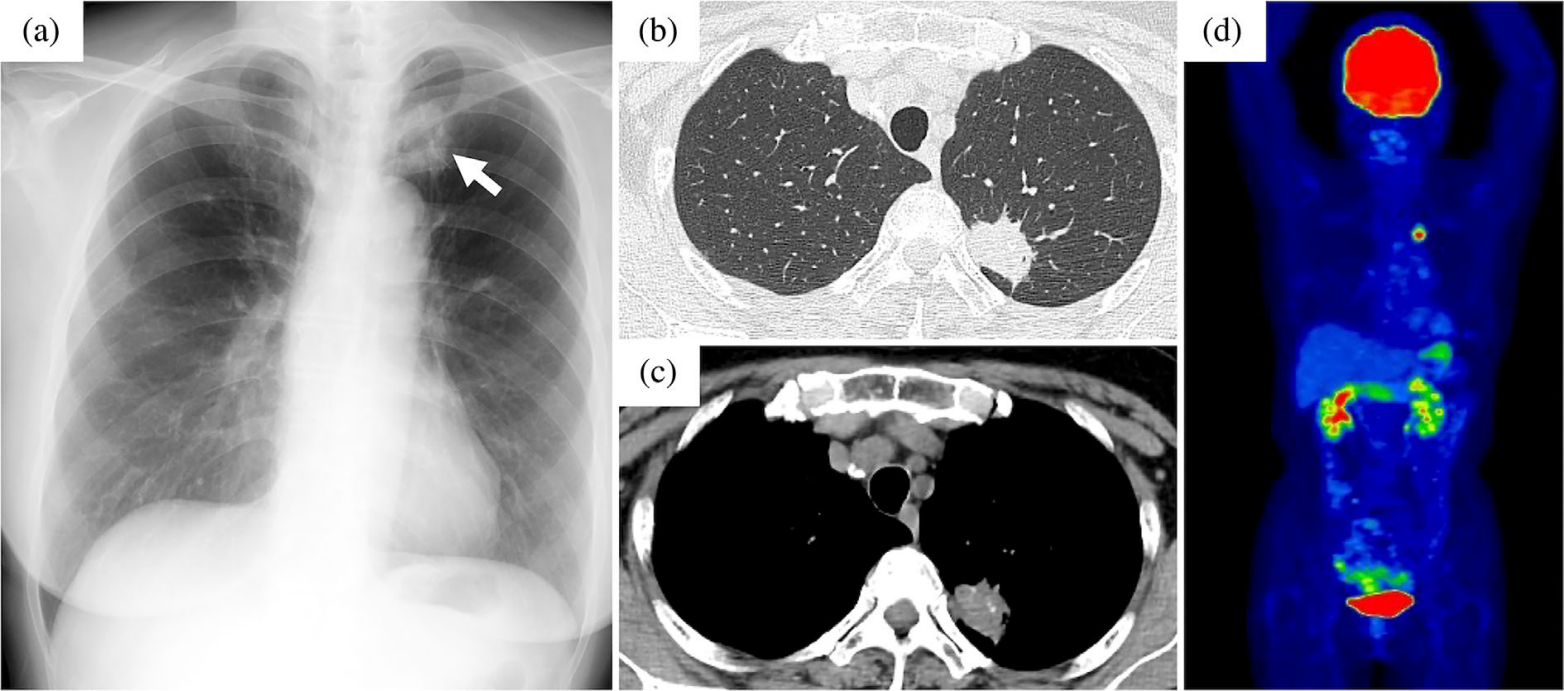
Chest x‐ray film on admission showed a tumor shadow in the left upper lung field (arrow) (a). Chest computed tomography (CT) on admission (b). CT showed a tumor with internal calcification in the left upper lobe (c). Positron emission tomography (PET) showed ^18^F‐ fluorodeoxyglucose accumulation consistent with a lung tumor (d).
